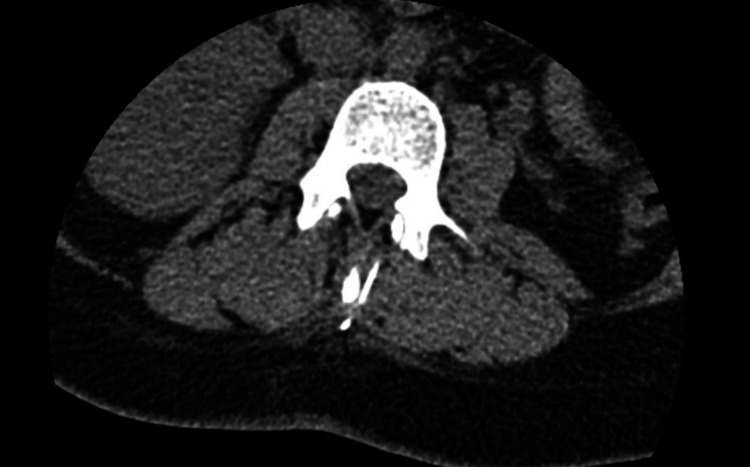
Axial CT scan at the L3 level showing the metallic fragment lodged in the paravertebral musculature, approximately 1 cm lateral to the spinous process.
